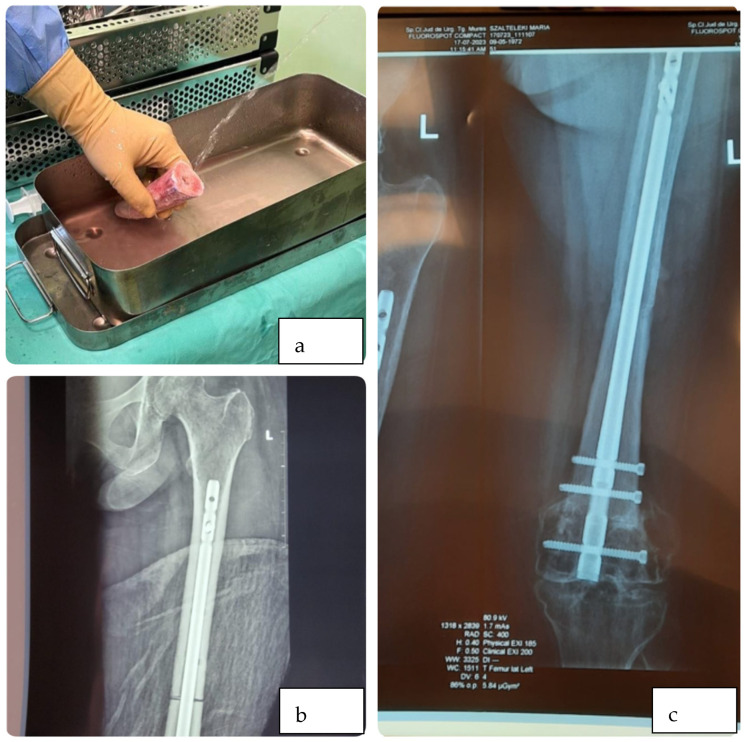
Case 3 (Part 2): Definitive reconstruction and progressive graft integration. (a) Intraoperative preparation and thawing of the structural nonvascularized bone allograft in warm saline in October 2022. (b) Postoperative radiograph showing the definitive construct stabilized with a retrograde intramedullary nail in October 2022. (c) Final follow-up radiograph demonstrating solid bridging callus and successful biological integration of the bone graft in February 2023.
